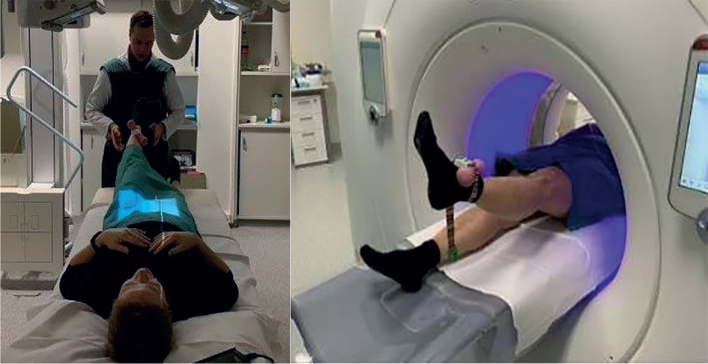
Setup with active straight leg raise during radiostereometric analysis (RSA) and CT-RSA.
